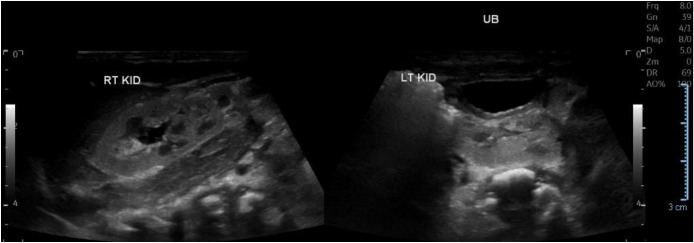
Initial postnatal renal ultrasound showing in situ right kidney with mild hydronephrosis, and a smaller left pelvic kidney, with preserved corticomedullary differentiation and normal parenchymal echogenicity (April 16, 2025).
